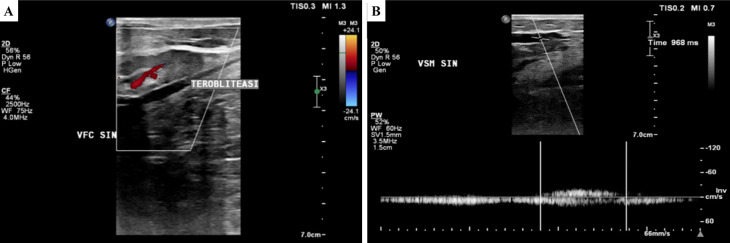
Lower extremity duplex ultrasound of the 2
